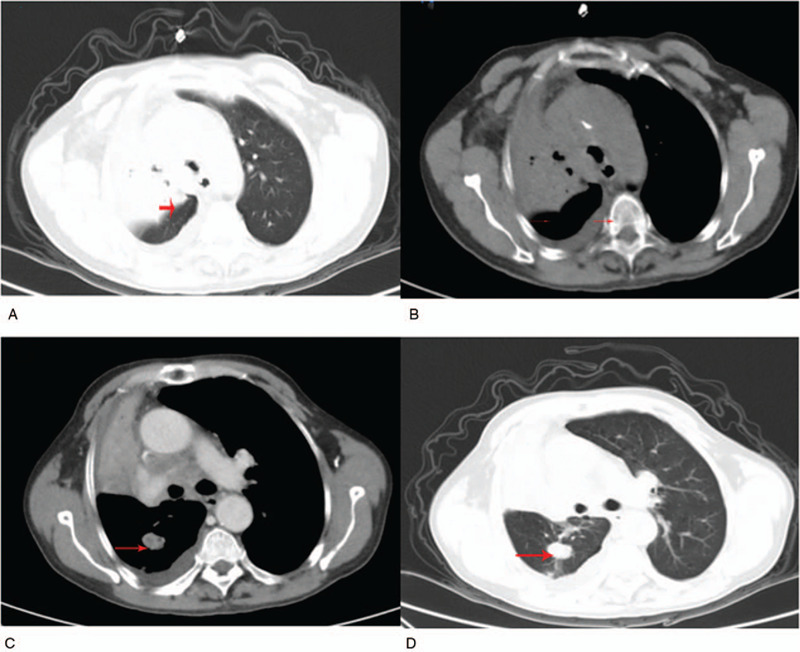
Computed tomography (CT): (A–D—axial CT images) results showed multiple patchy opacities in the anterior segment of the right upper lobe, left lower lobe, and lingual segment of left upper lobe along with multiple para-mediastinal and right hilar lymph nodal enlargements.
